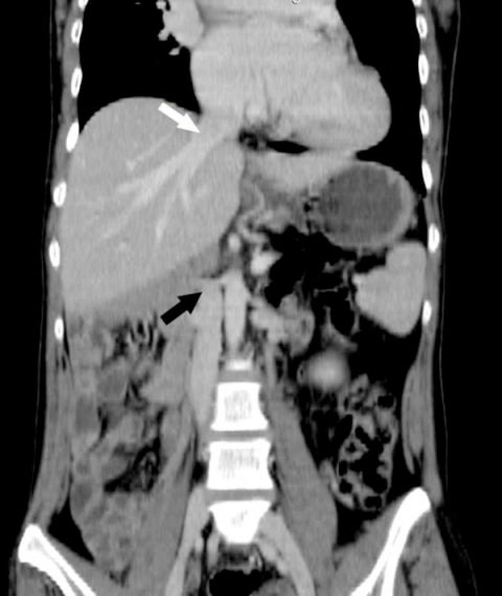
Coronal multiplanar reformation CT image demonstrates the absence of inferior vena cava (black arrow) and hepatic veins draining directly into the right atrium (white arrow).
